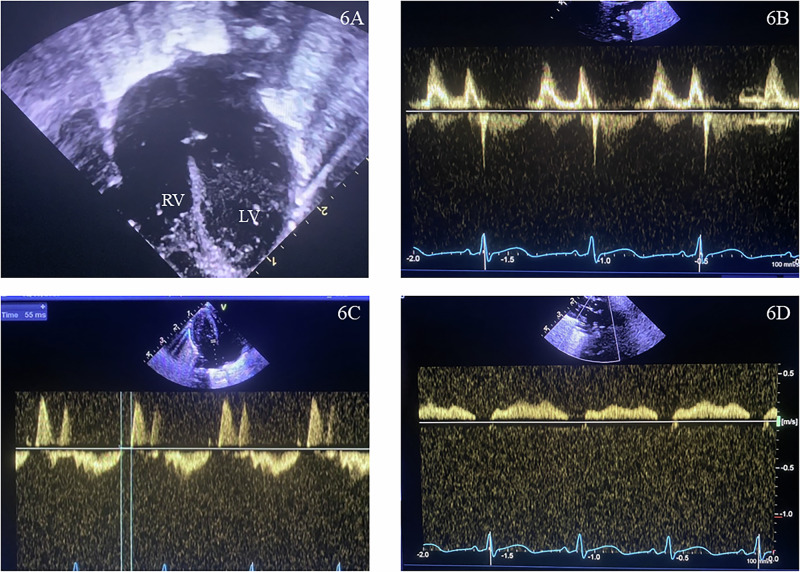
Pre-captopril echocardiographic information. A Left ventricular dilatation on 2D apical 4 chamber view. B Reversed trans-mitral E/A pulse Doppler. C Iso-volumic relaxation time. D Dampened pulmonary venous Doppler.
